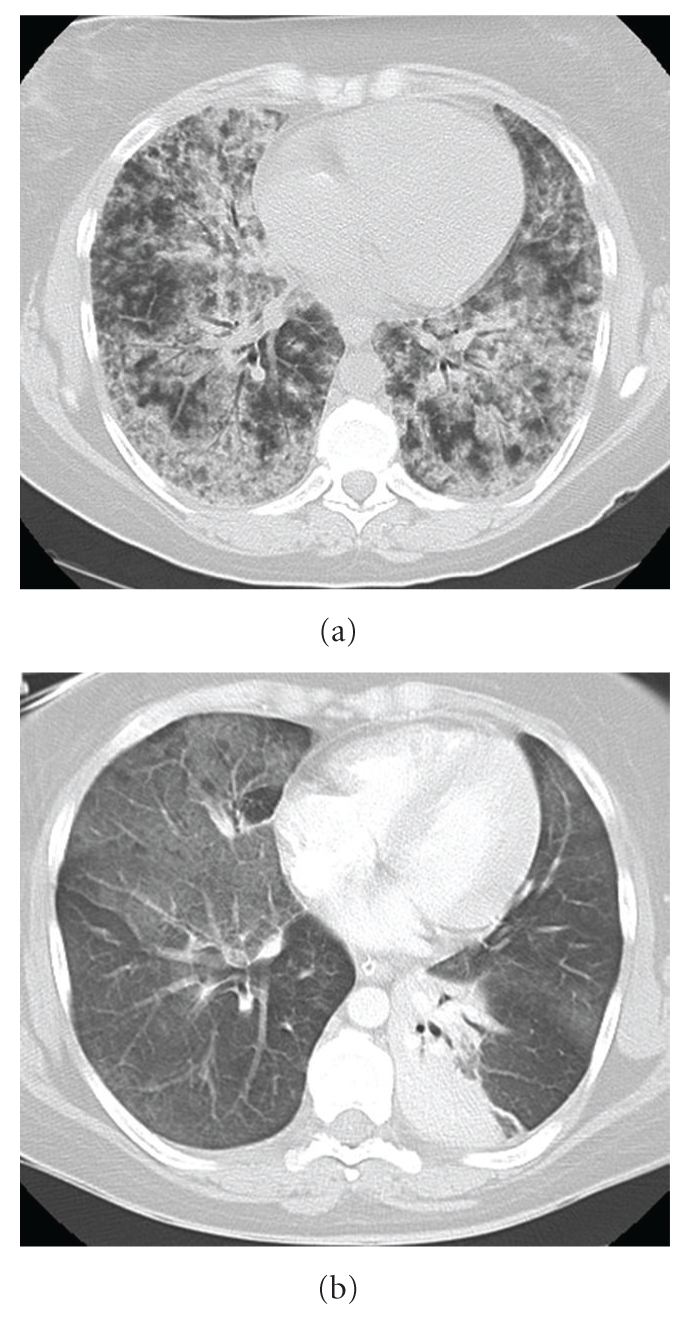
(a) CT scan of the chest obtained on day 1 showing diffuse alveolar infiltrate. (b) CT scan of the chest obtained on day 8: right ground glas opacities and atelectasis of the left lower lobe.
